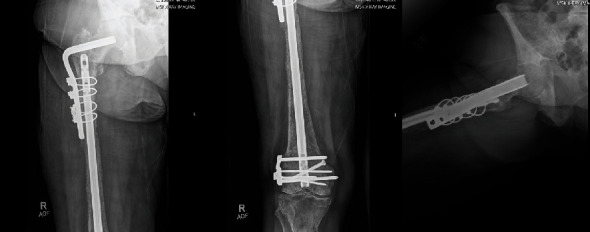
Two-month postoperative radiographs demonstrating healing of previous pathologic peri-implant femur fracture without concern for hardware failure.
